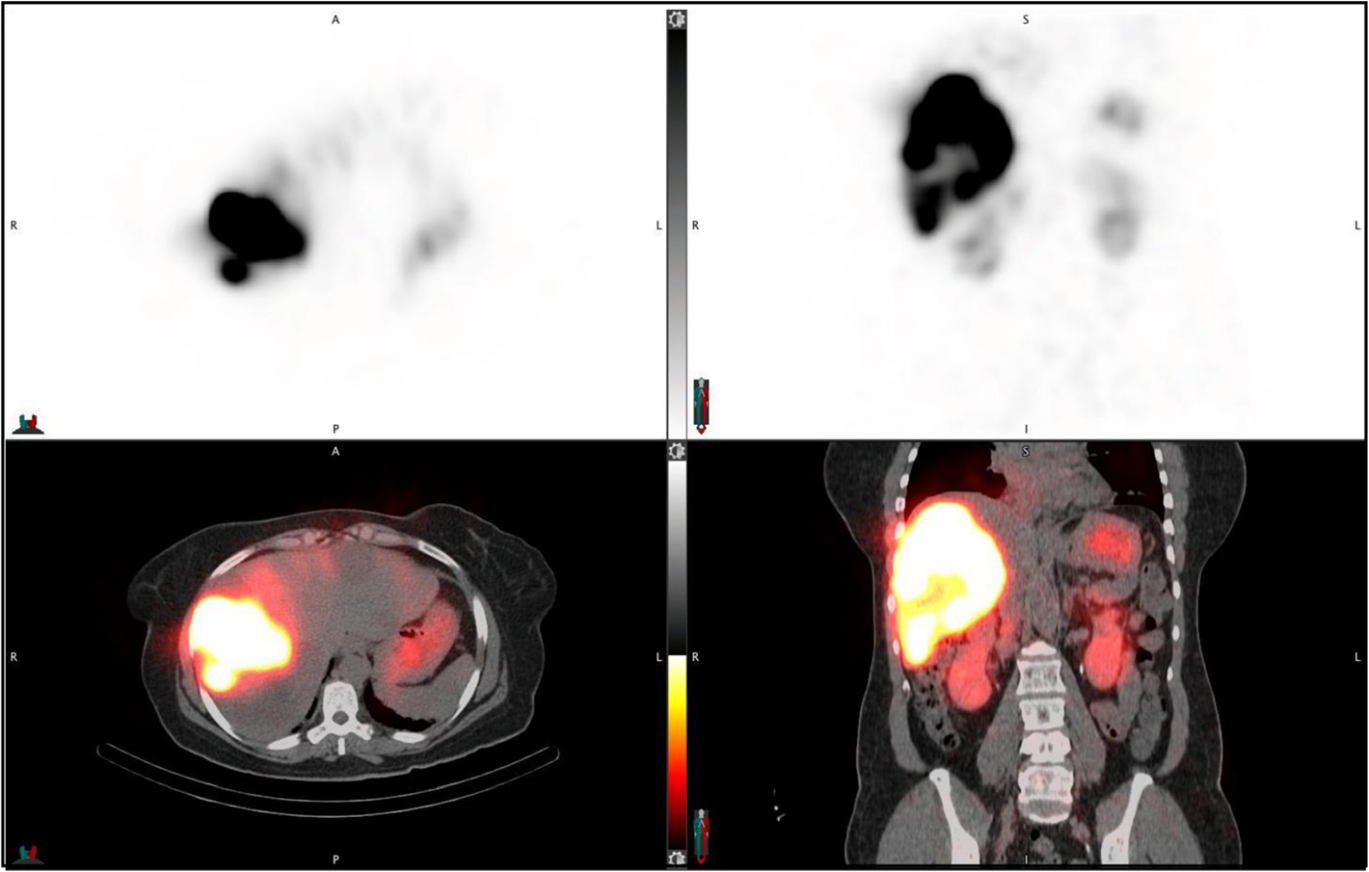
^99m^Tc-MAA SPECT/CT images corresponding to a patient where the time between injection and imaging was 176 min demonstrating gastric mucosal and renal cortical extra-hepatic uptake
